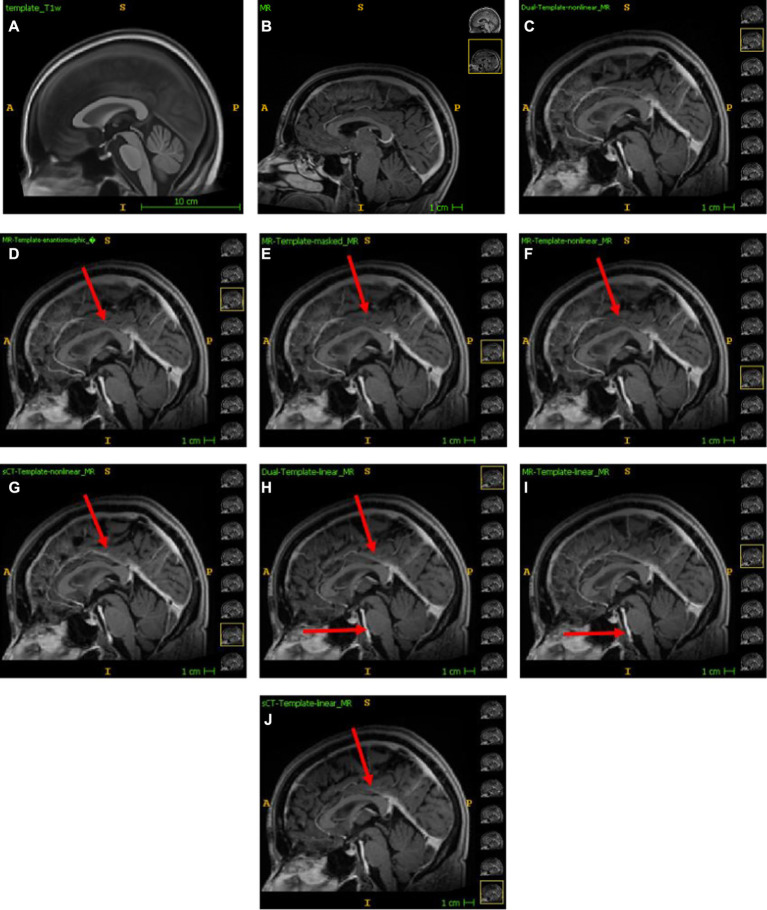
Comparison of sagittal slices of the MR images registered on to the template space (A), the native space (B) using the Dual-Template-non-linear (C), MR-Template-enantiomorphic (D), MR-Template-masked (E), MR-Template-non-linear (F), sCT-Template-non-linear (G), Dual-Template-linear (H), MR-Template-linear (I), and sCT-Template-linear schemes(J). The images are shown from highest to lowest score, with the Dual-Template-non-linear scoring a 5 and the sCT-Template-linear scoring a 2. The red arrows point to distortions in the corpus callosum and brainstem.
